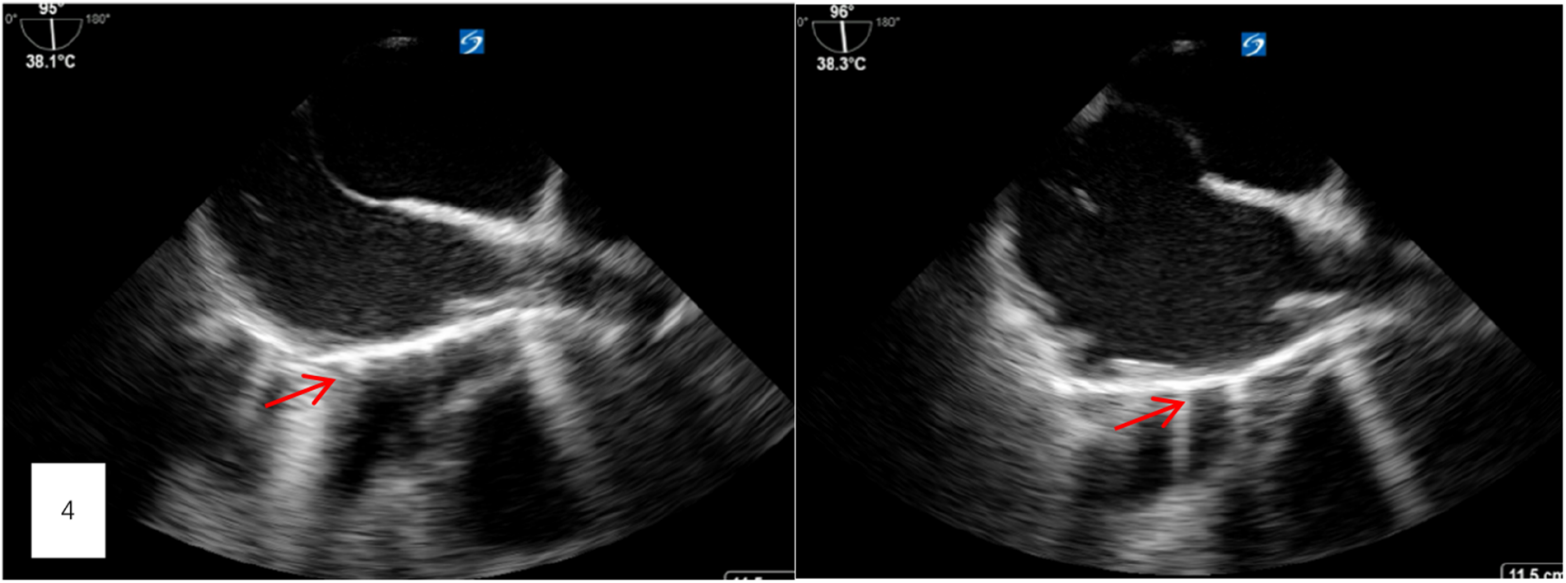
Preoperative transesophageal ultrasound, conducted after general anesthesia, confirmed the presence of a foreign body in the right atrium, measuring approximately 5 cm in length.
